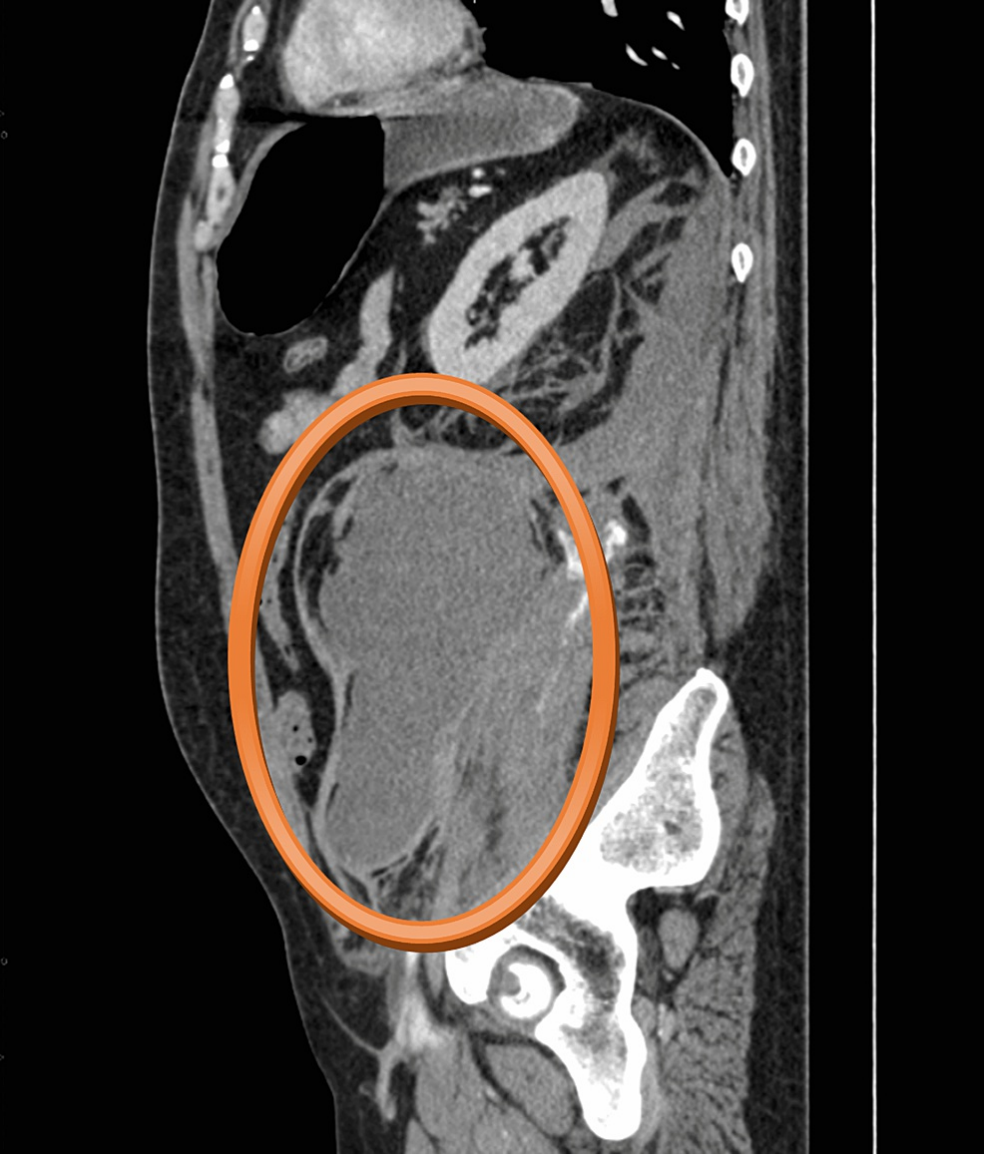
CT scan - sagittal plane The hematoma in the left retroperitoneum was of size 170 x 125 x 120 mm, attached to the left musculus psoas major, with present active bleeding
